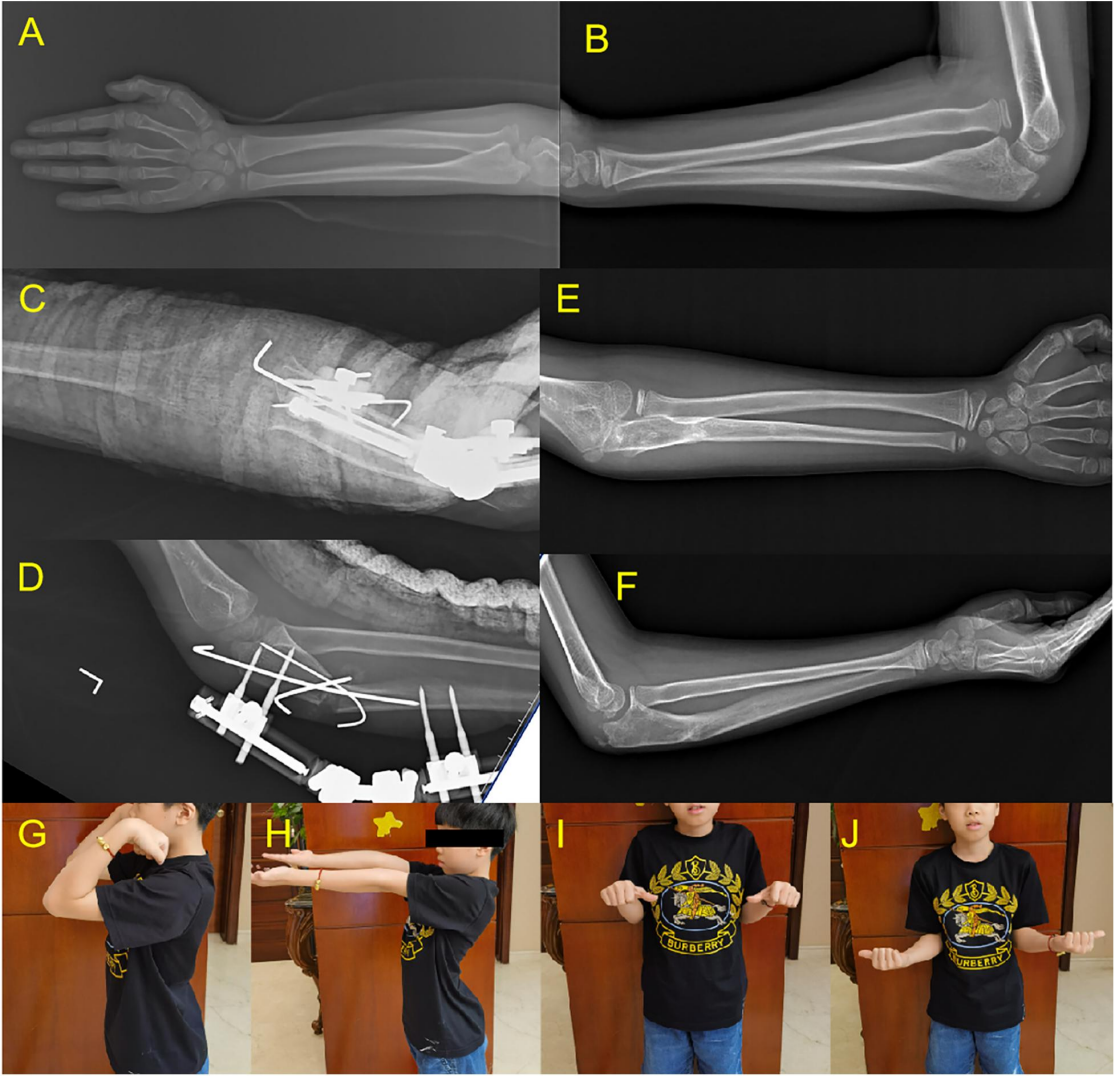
An 8-year-old male with left arm injury 12 months prior. (A,B) Preoperative X-rays showing anterior dislocation of the radial head. (C,D) Postoperative X-rays showing ulnar osteotomy with lengthening and angulation, fixed with external fixator, K-wires, and plaster slab. (E,F) X-rays at 1-year follow-up showing well-healed ulna and maintained humeroradial joint reduction. (G–J) Normal elbow flexion-extension, pronation, and supination at 1-year follow-up.
